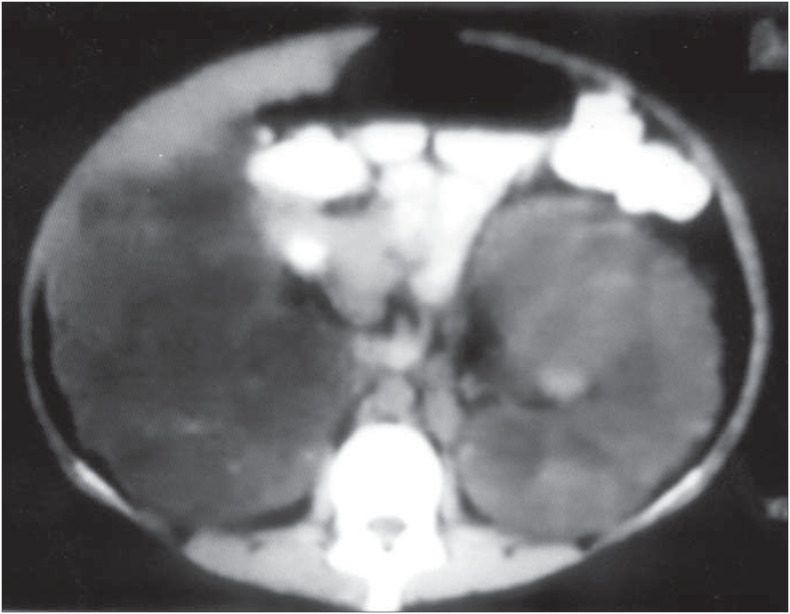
Appearance of the kidneys via computed tomography scan.
